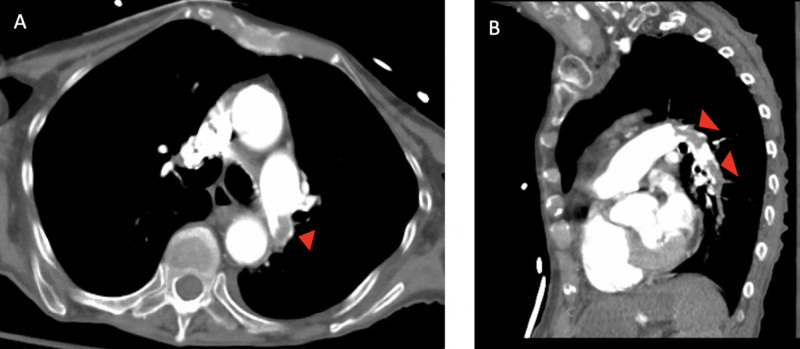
Computed tomography pulmonary angiogram demonstrating an embolus in the left distal pulmonary artery (arrowheads). (A) Axial view. (B) Sagittal view.
